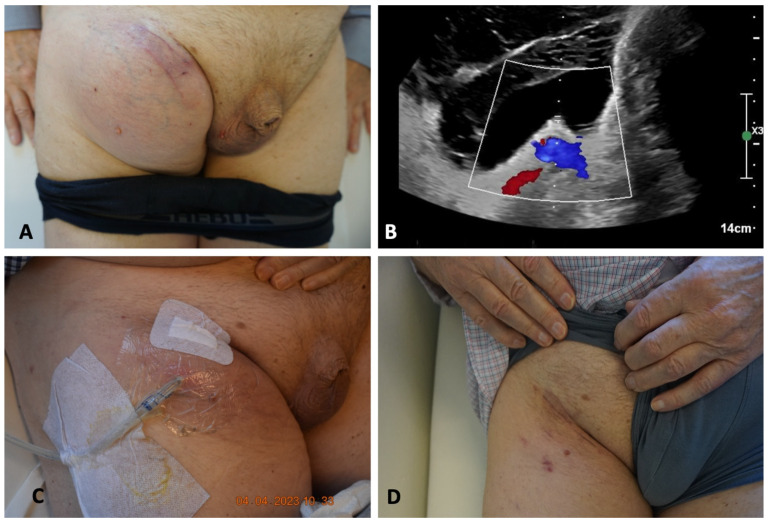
(A): Before surgery. (B): Ultrasound imaging of the lymphocele above the femoral vessels before HV-LVA. (C): After insertion of a drain in local anesthesia. (D): 3 months after HV-LVA (SCIV).
